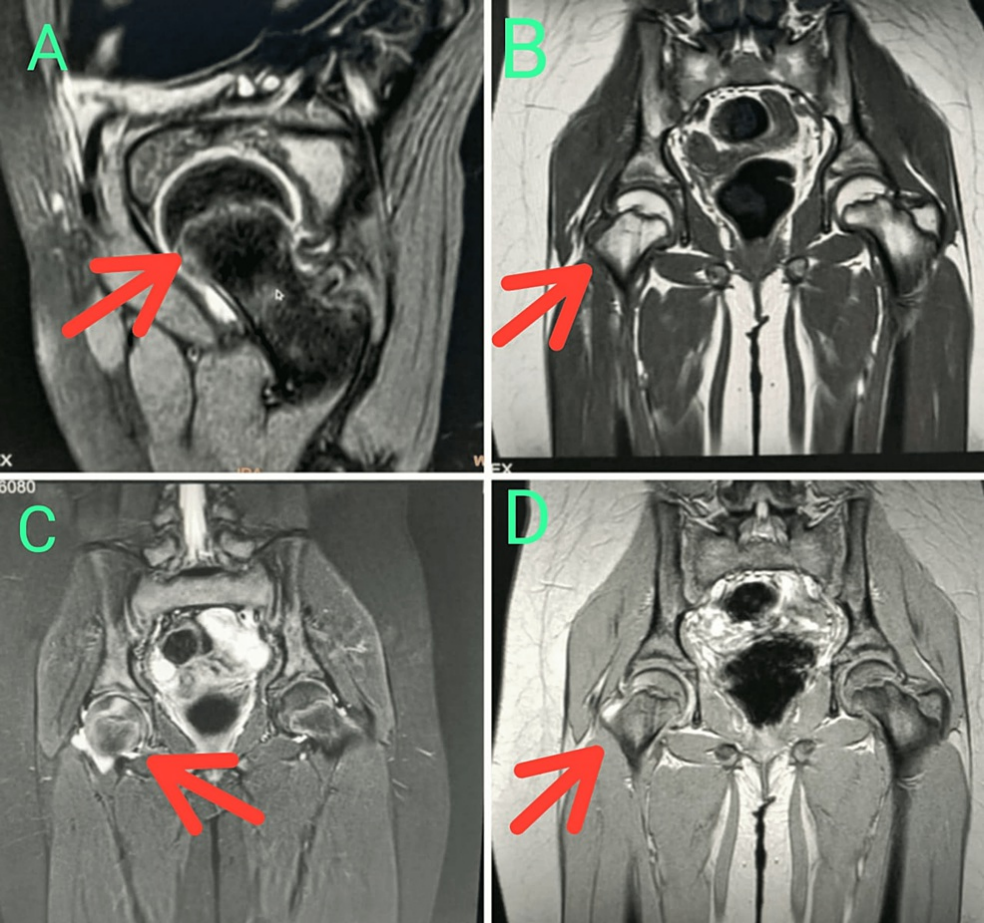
MRI of the hip regions at the time of presentation. (A, B) T1 weighted, (C, D) T2 weighted Focal marrow oedema (red arrows) in the right femoral epiphysis with minimal joint effusion. Possibility of idiopathic chondrolysis of hip.
